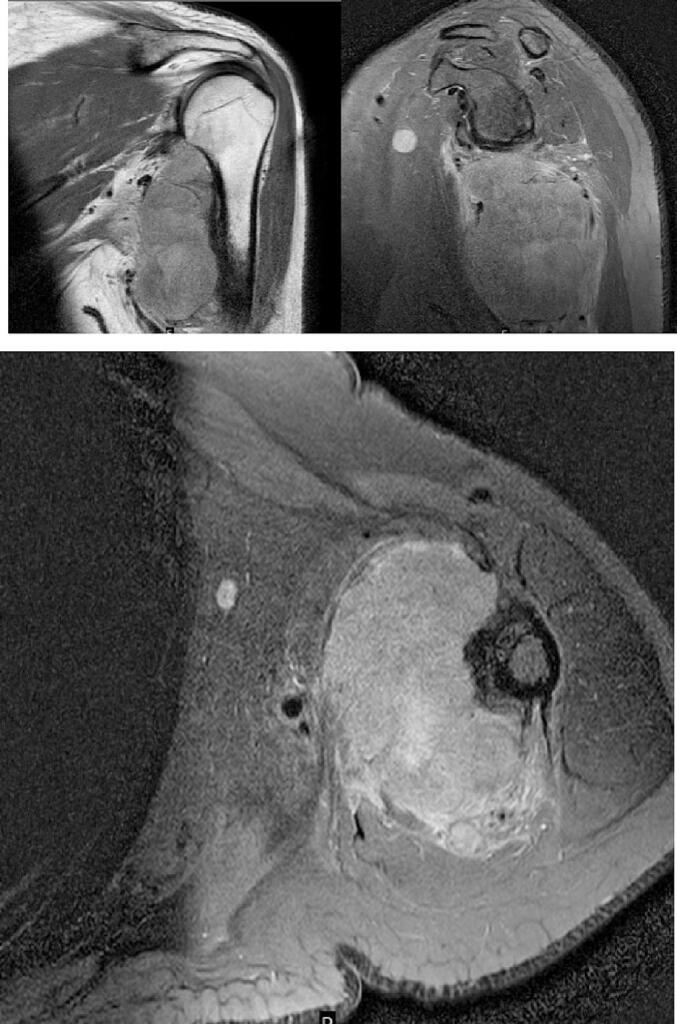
MRI of Left Shoulder: a dense soft tissue mass, lobulated, and indefinitely demarcated with size approximately 5,6 cm × 7,8 cm × 8,8 cm.
